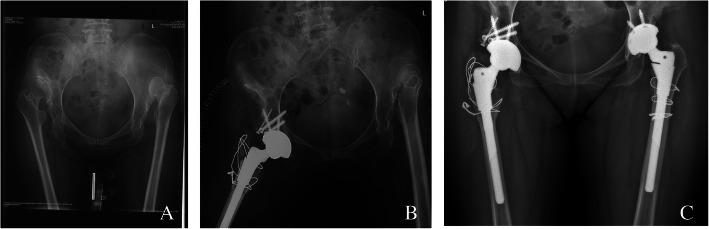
a Patient 20,Preoperative x-ray. b X-ray after operation 3 days.The femoral tuberosity was split during the operation and tied with steel wire. c X-ray after 2 year, the fracture heals
